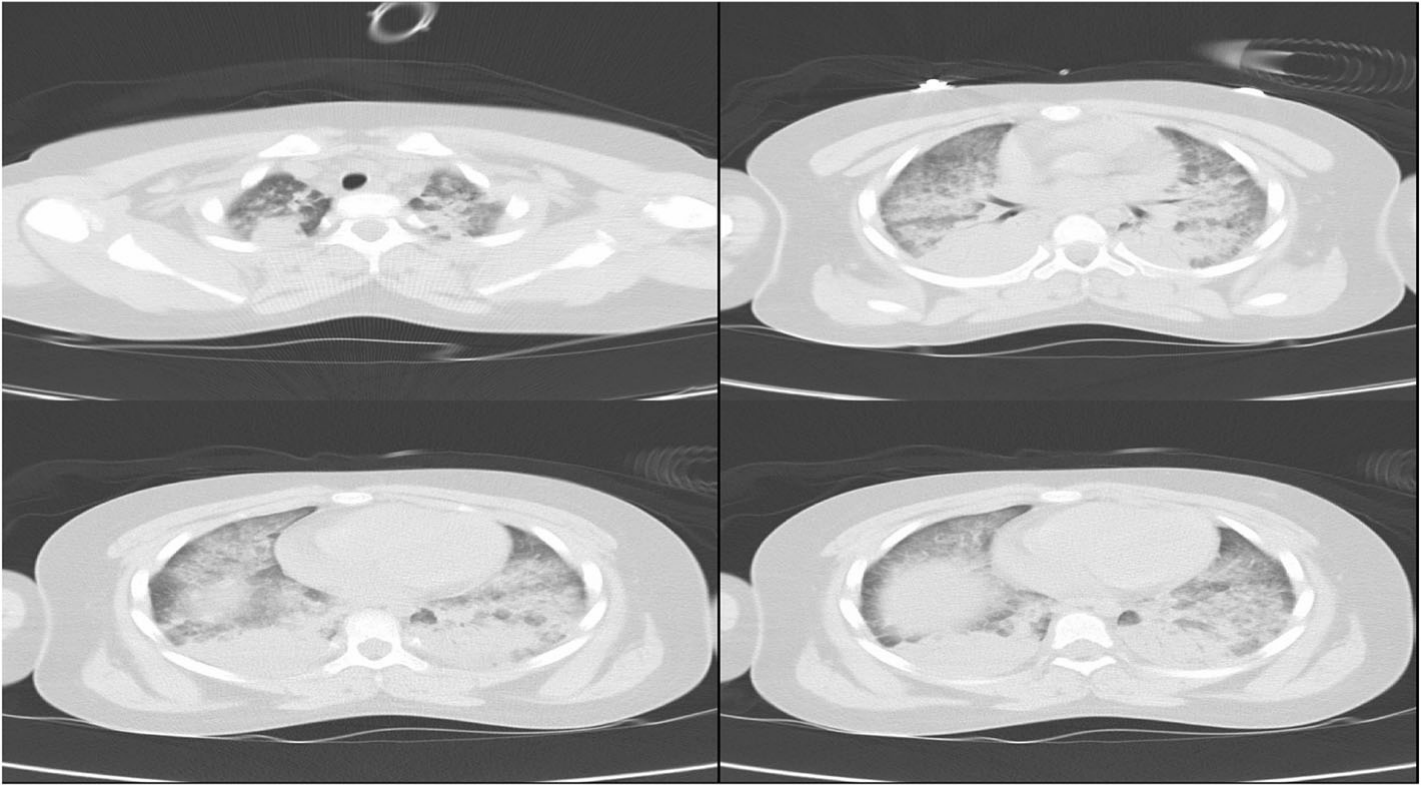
Chest CT of 4th April (The day of operation). These images demonstrated bilateral pulmonary consolidation accompanied by air bronchogram and the ground-glass opacity lesions
